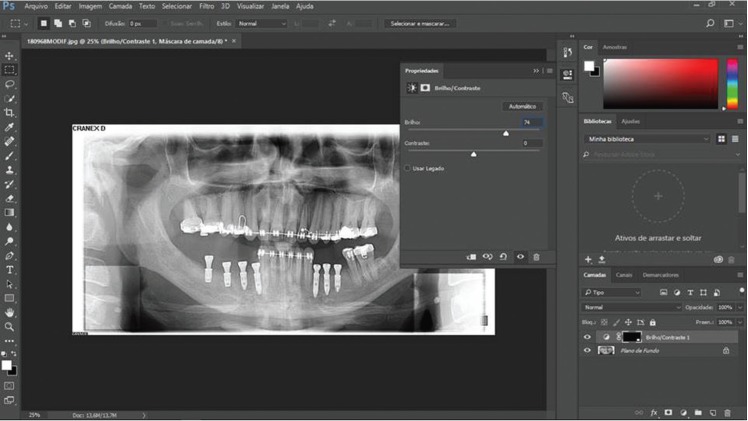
Adobe Photoshop® window showing the use of a filter with saturation and balance standardized in order to analysis a bilateral CAC on panoramic radiograph.
